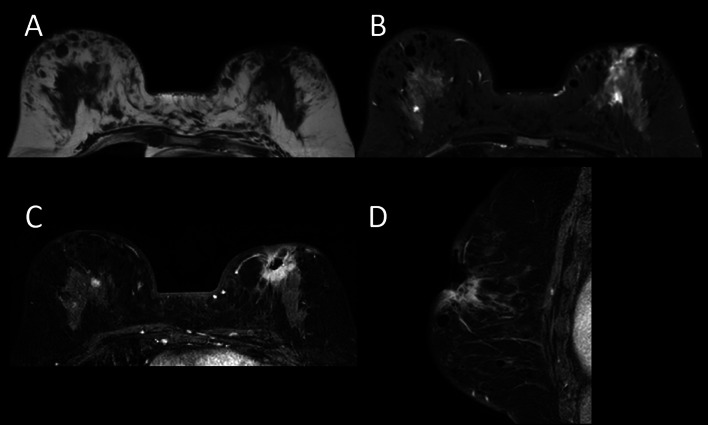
Breast MRI. A Axial T1WI B Axial fat suppressed T2WI C Axial contrast enhanced T1WI. D Sagittal contrast enhanced T1WI. Breast MRI shows a large ulcerative lesion in the left breast (arrow). There is an irregular shaped enhancing mass on the base of the ulcer and it is continuous with the mammary gland tissue (C and D). Many subcutaneous nodules are also seen in the both breasts. The T1-weighted image and fat-suppressed T2-weighted image (B) show no signal in those nodules (arrowhead). These are consistent with the liquid silicone materials injected before
